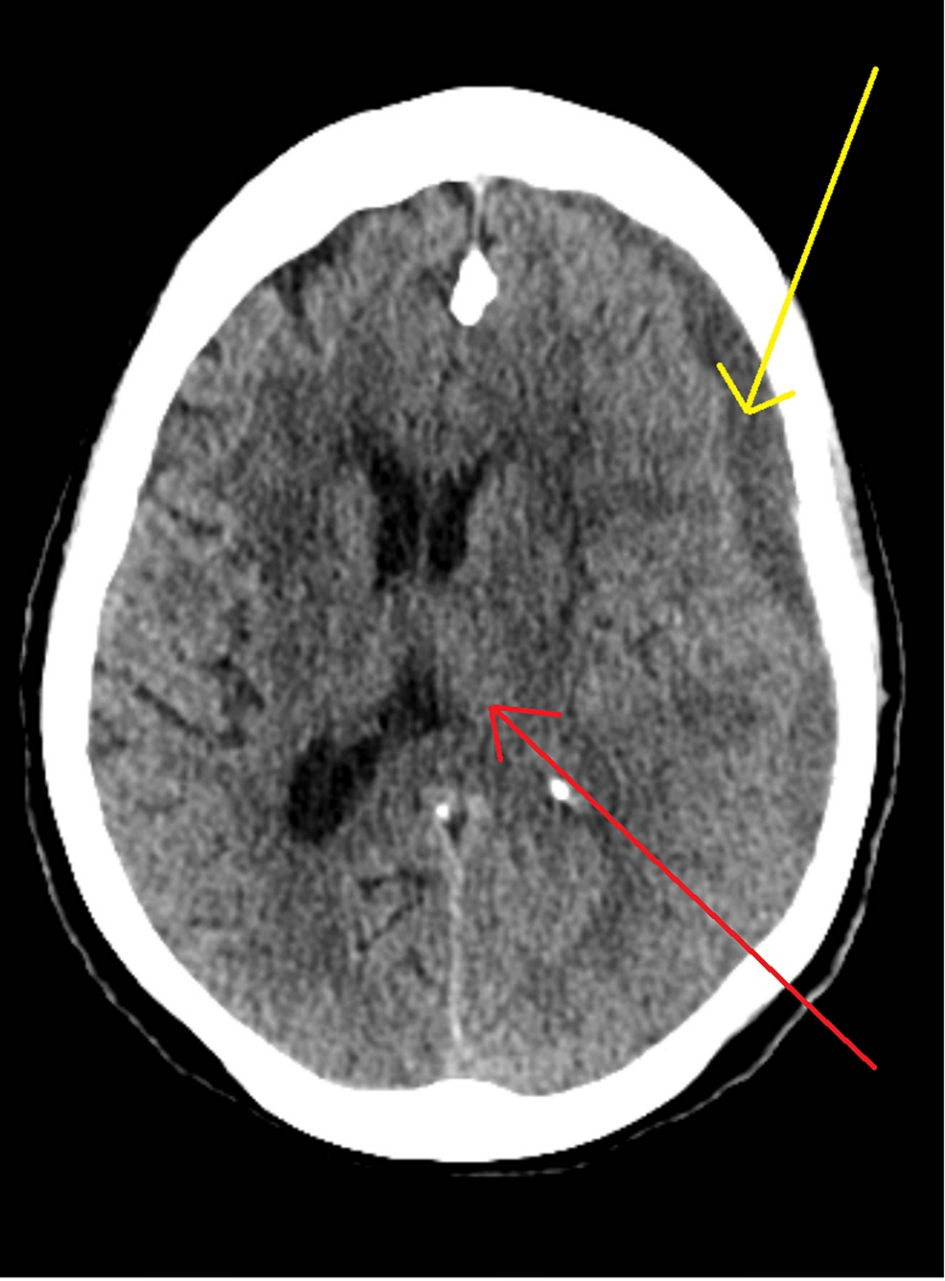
Axial view of computed tomography of the brain showing chronic subdural hematoma (yellow arrow) and herniation with midline shift (red arrow).
